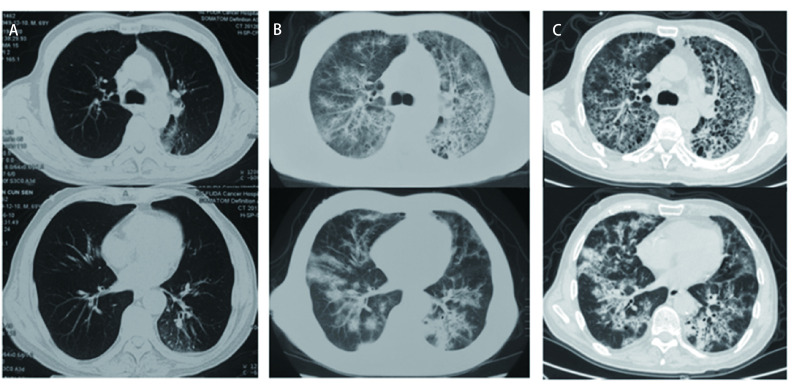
CIP发病前后肺部CT。A：第6周期纳武利尤单抗治疗后胸部CT（2019年6月10日）：表现为肺气肿、肺大泡和局限性淋巴管炎；B：第8周期纳武利尤单抗治疗后胸部CT（2019年10月5日）：表现为弥漫的磨玻璃样影伴网格状改变以及沿支气管分布的结节状斑片状实变影；C：第9周期纳武利尤单抗治疗后胸部CT（2019年10月28日）：表现为弥漫的磨玻璃样影伴网格状改变以及沿支气管分布的结节状斑片状实变影。 Pulmonary CT before and after the onset of CIP. A: Chest CT after treatment with Nivolumab in cycle 6 (June 10, 2019): emphysema, pulmonary bullae and localized lymphangitis; B: Chest CT after treatment with Nivolumab in cycle 8 (October 5, 2019): diffuse ground glass opacity with grid like changes and nodular patchy consolidation along the bronchus; C: Chest CT after treatment with Nivolumab in cycle 9 (October 28, 2019): diffuse ground glass opacity with grid like changes and nodular patchy consolidation along the bronchus. CIP: immune checkpoint inhibitor associated pneumonia; CT: computed tomography.
